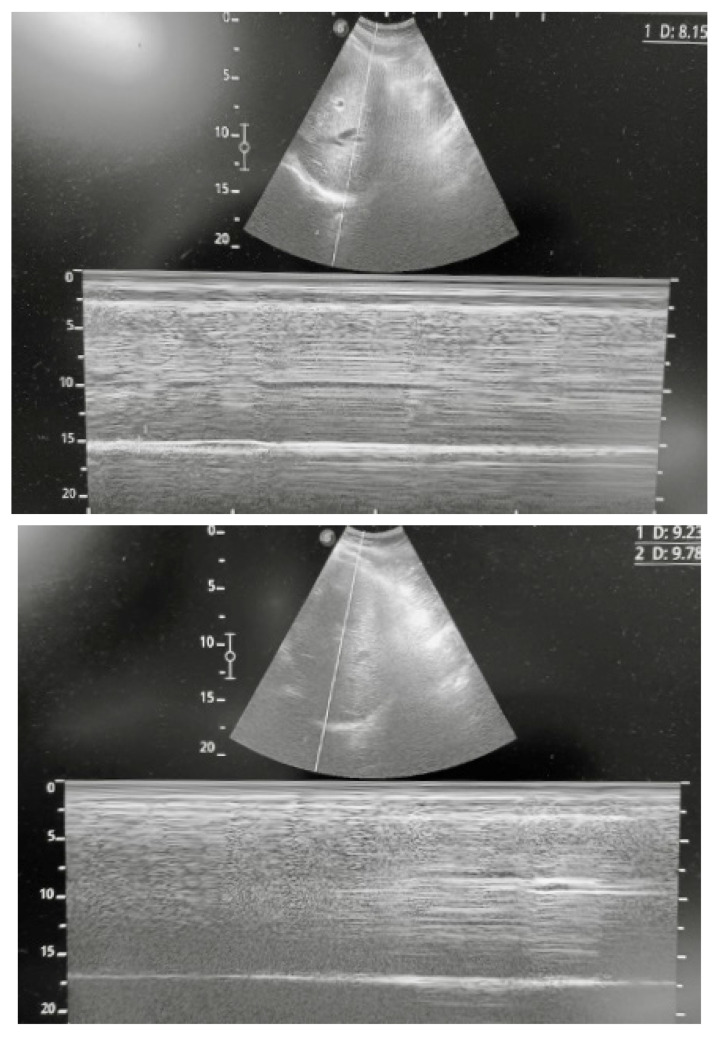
Ultrasound evaluation of B.M.’s right hemidiaphragm during resting breathing; upper image, end-exhalation, lower image, end-inhalation. B-mode and M-mode were used for the evaluation of the diaphragm excursion: it can be easily noted that there were no diaphragm movements during resting breathing.
